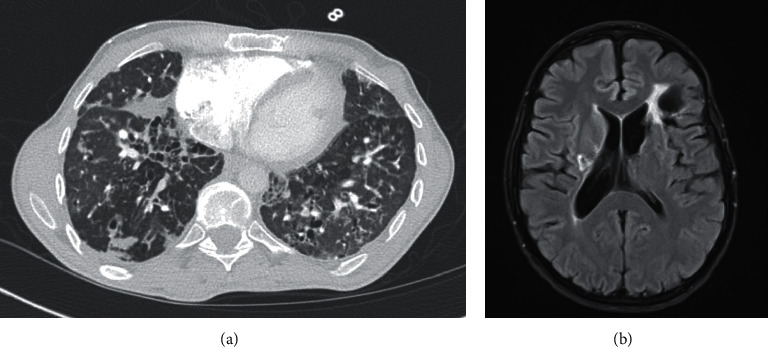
Radiology at age 43. (a) Chest CT scan with diffuse consolidations, bronchiectasis, and cysts. (b) MRI brain showing bilateral areas of acute infarction and left frontal encephalomalacia from prior insult.
